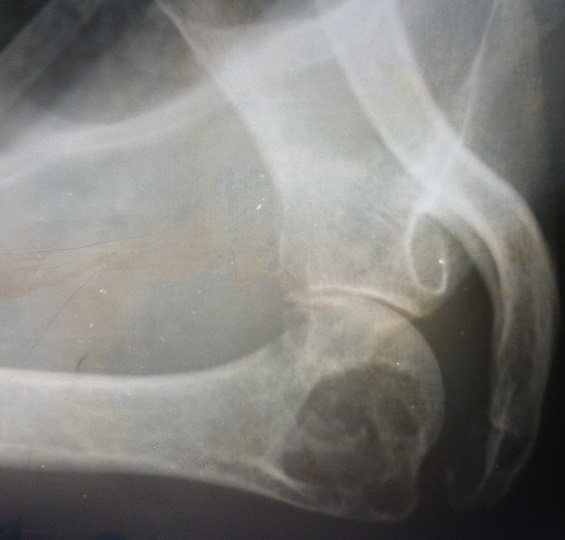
Radiographie de l’épaule gauche de face: pincement de l'interligne, aspect hétérogène, ostéolyse, géodes, de la tête humérale
